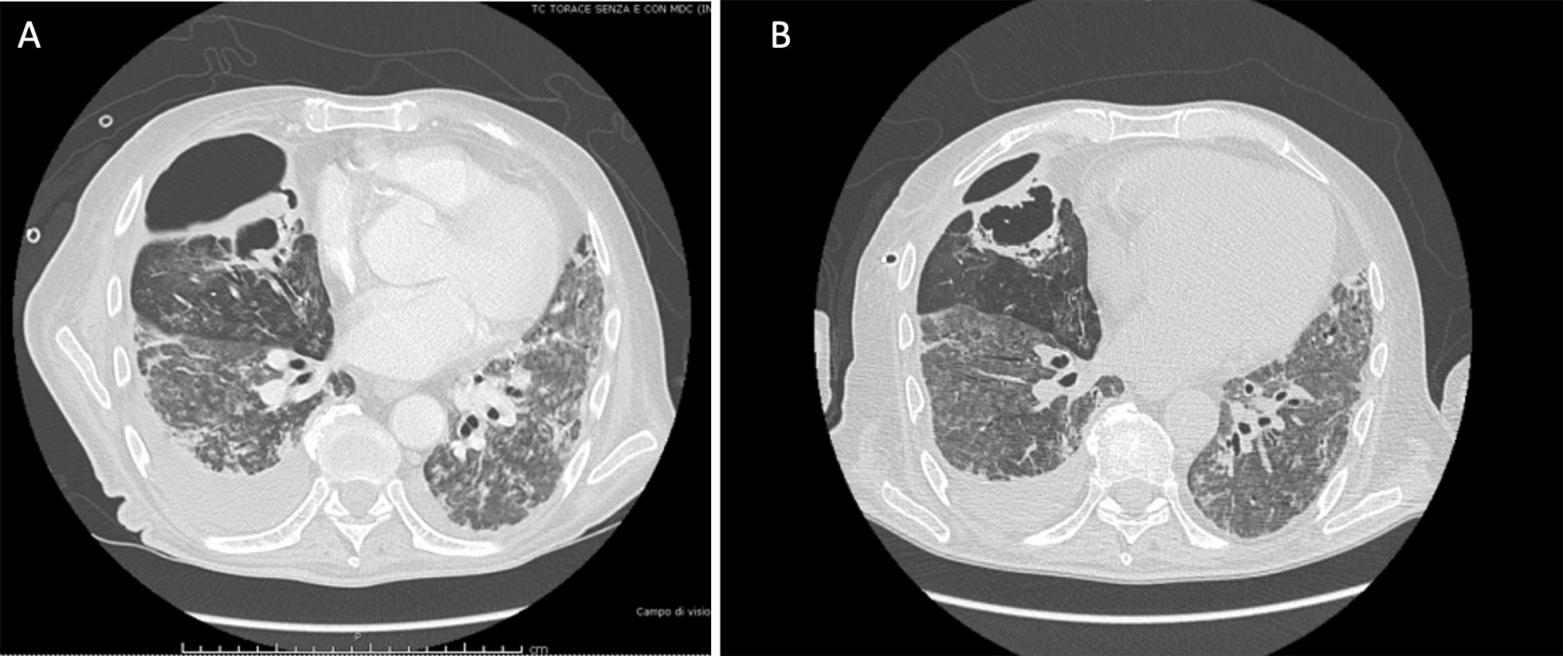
High resolution computed tomography scan in Case 1 showing right pneumothorax secondary to alveolar-pleural fistula (APF) before (panel A) and after (panel B) endobronchial valve (EBV) positioning with lung parenchyma re-expansion
